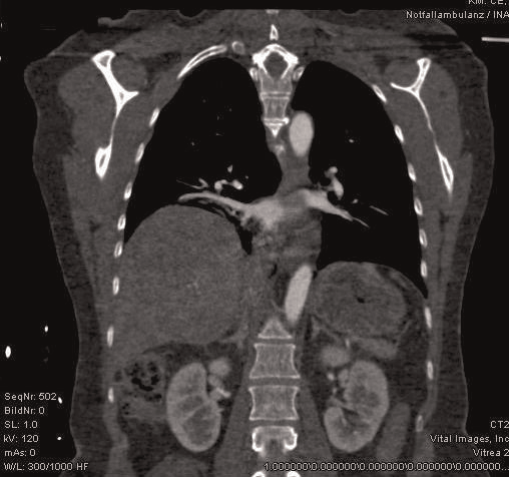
Reconstruction of the CT-scan: full displacement of the liver into the right thorax.
